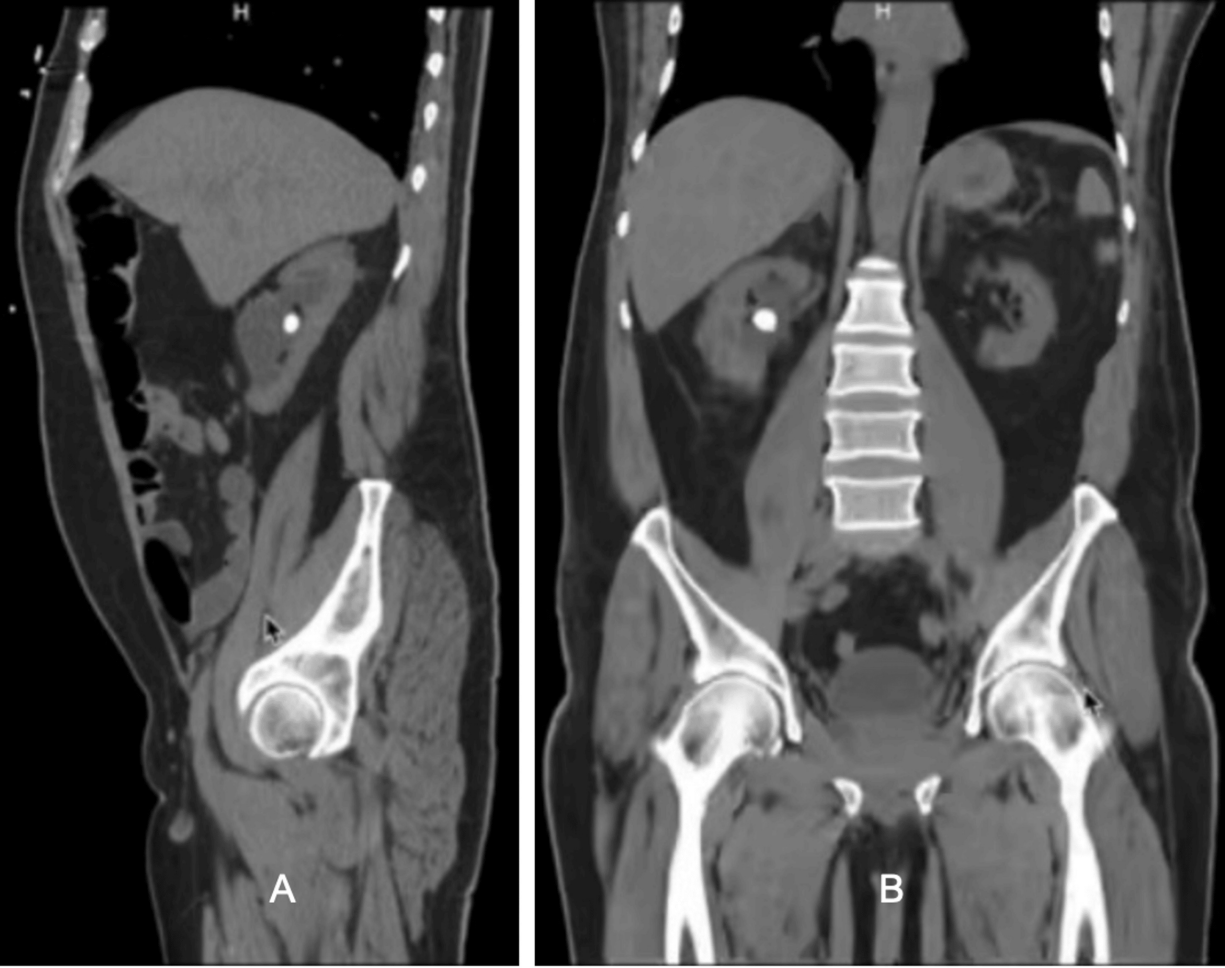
A- Sagittal view; B- Coronal view. Both panels A and B show CT abdomen + pelvis with non-acute urologic finding of probable kidney stone.
